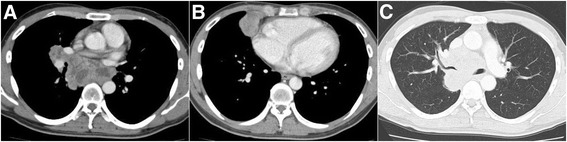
Full-body CT scan at the first visit. Tumor in the mediastinum and lymphadenopathy in the right side of the hilum and supraclavicular region (a). A tumor in the right middle lobe (b). Right bronchial stenosis due to the mediastinal tumor is shown (c)
